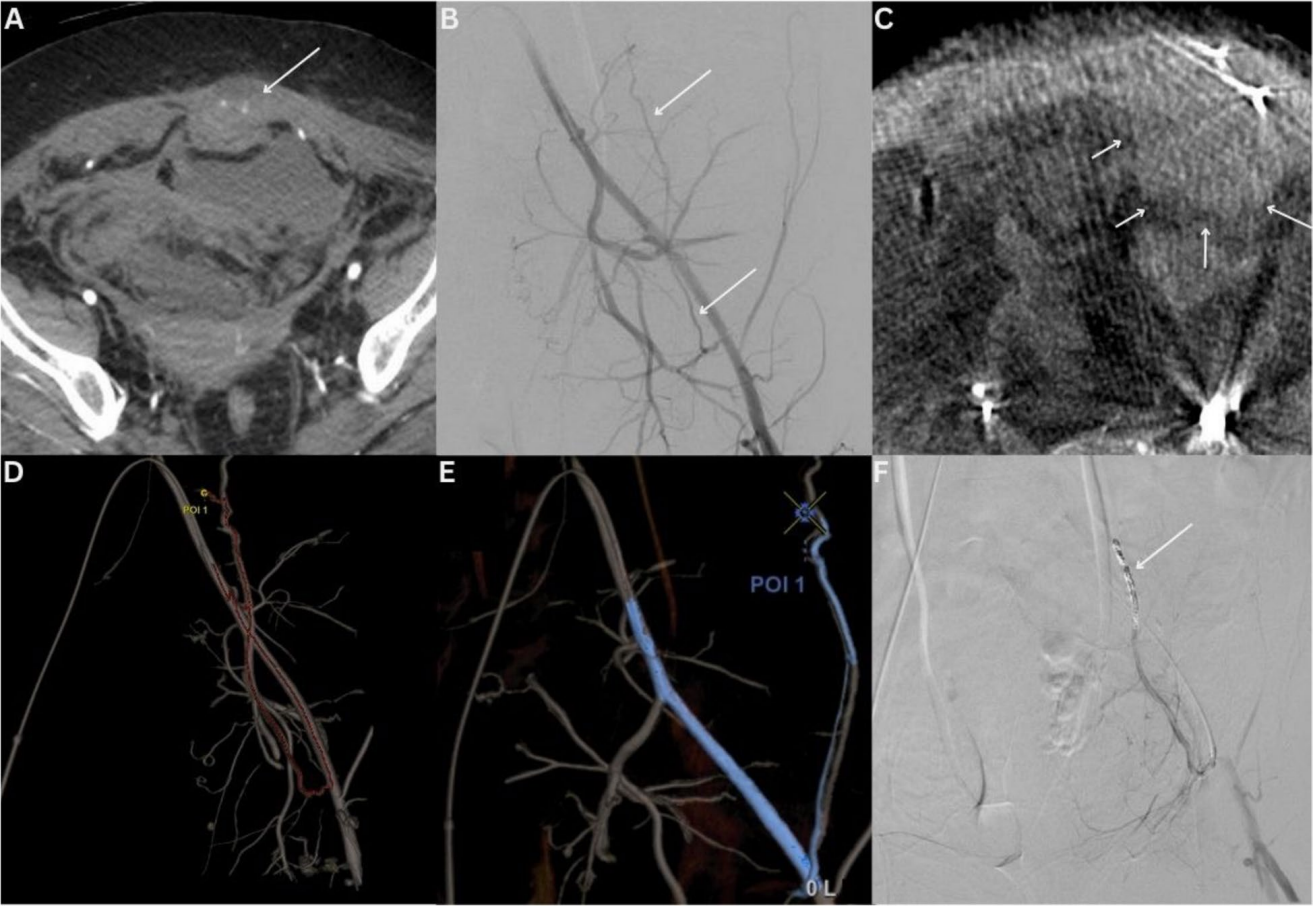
Case 2—Inferior epigastric artery embolization A 50-year-old woman presenting with bleeding into the left rectus abdominis. A Foci of contrast extravasation (arrow) noted on the patient’s pre-procedural CT Angiogram. B No definitive focus of extravasation seen from the left inferior epigastric artery (IEA–white arrows) on left iliac artery DSA. C Intra-procedural CBCT confirmed no evidence of contrast extravasation but an increase in size of the rectus sheath hematoma (white arrows). D The arterial tree was automatically segmented and the point of interest (correlating with the level of abnormal IEA noted on the positive pre-procedural CT) was selected with automatic path calculation from the desired proximal position (Embo ASSIST with Virtual Injection, GE HealthCare, Chicago, IL, USA). E A colored three-dimensional roadmap was then generated from the processed data. F Post-coil embolization left EIA DSA demonstrates successful post-embolization appearances
